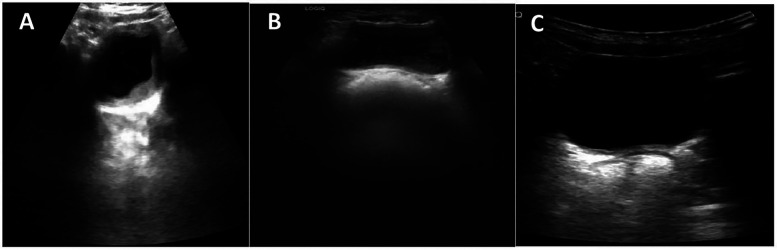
Ultrasonographic images of the bladder at different time points. (A) Bladder ultrasound on the first day of admission, showing sediment in the bladder. (B) Follow-up bladder ultrasound performed two weeks post-discharge, revealing there's still visible bladder sediment. (C) Follow-up bladder ultrasound one month post-discharge, showing the bladder sediment disappeared.
